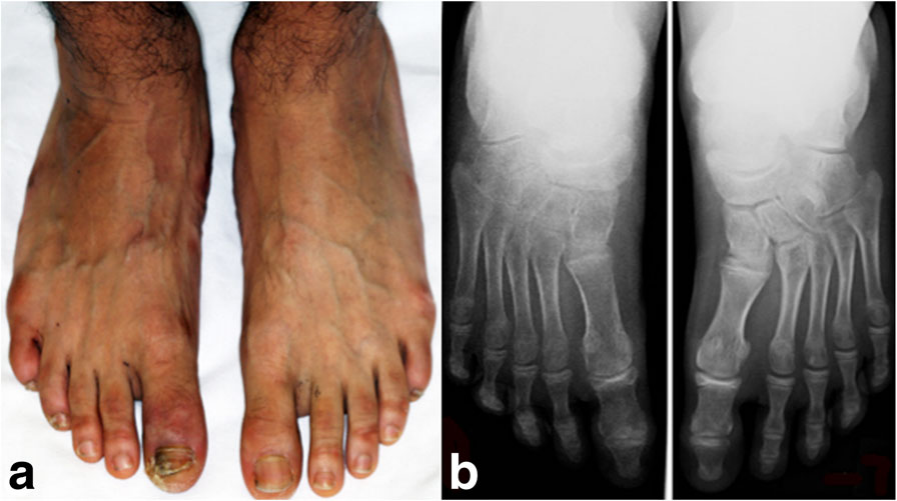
Bilateral bone deformity of the feet. a Clinical presentation of the deformed feet. b Radiographic presentation of the deformed feet
